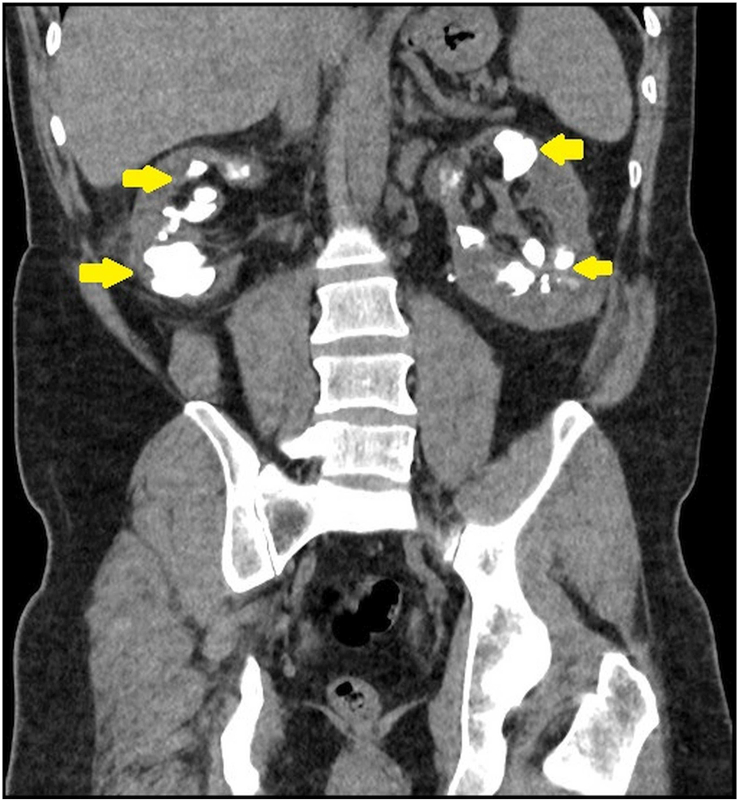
Non contrast computed tomography of the abdomen and pelvis, coronal plane. There are bilateral dilated renal calcyes, each filled with calculi. A semilunar shape of the calyces along with their high number compared to a normal kidney can be seen. Renal parenchyma appears to be conserved in contrast to classical staghorn calculi.
